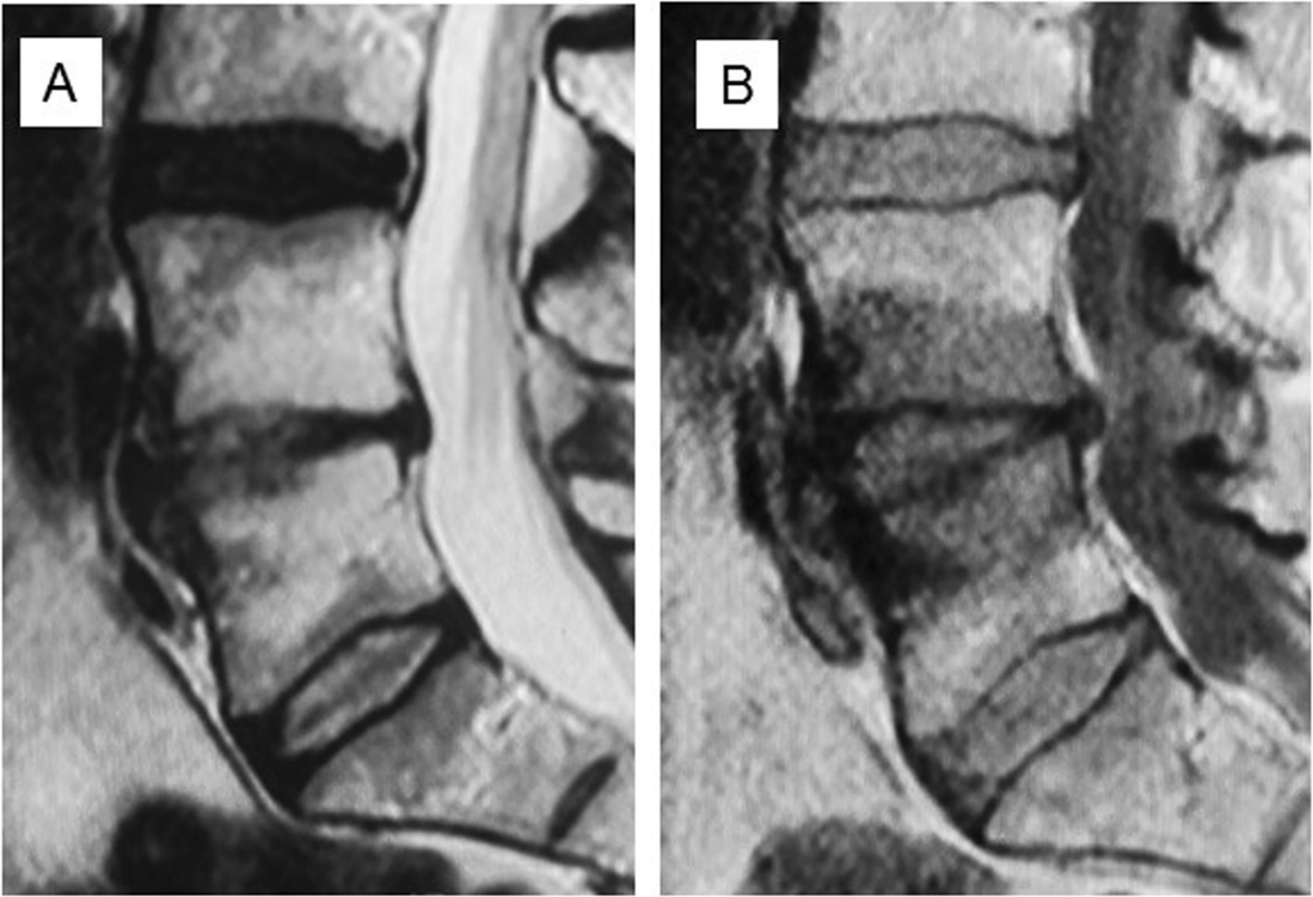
Baseline T2 (A) and T1 (B) -weighted sagittal MR images of a 45-year-old man with Type 1 Modic change at L4/5. Low back pain intensity 8/10, Oswestry Disability Index 24%.
